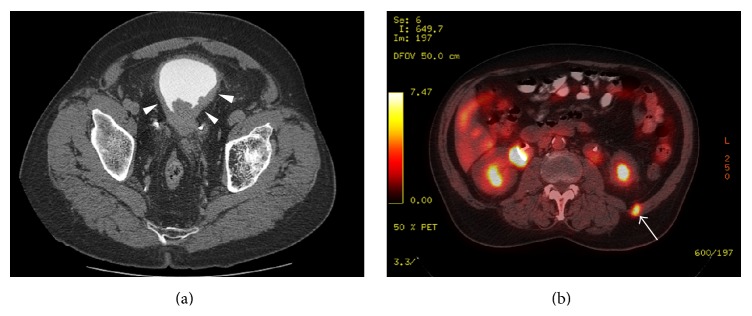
Imaging studies: (a) CT of the abdomen and pelvis showing diffuse bladder wall thickening (*arrowheads*) with perivesical fat stranding, indicating involvement of the bladder by invasive carcinoma; (b) PET of the abdomen and pelvis showing a 1 cm FDG-avid nodule in the musculature of the left flank, suspicious for metastasis (*arrow*).
